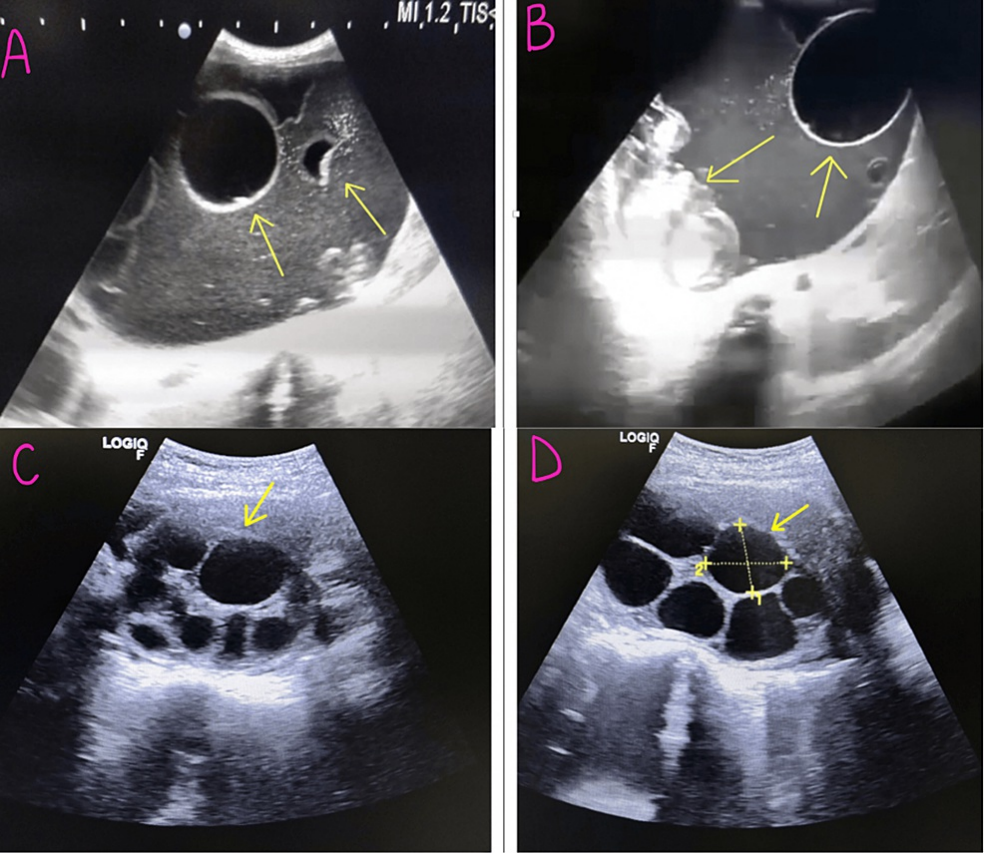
A & B: Ultrasonographic image of liver hydatid cyst showing unilocular cyst; C & D: Hydatid cyst of adnexa showing daughter cysts
